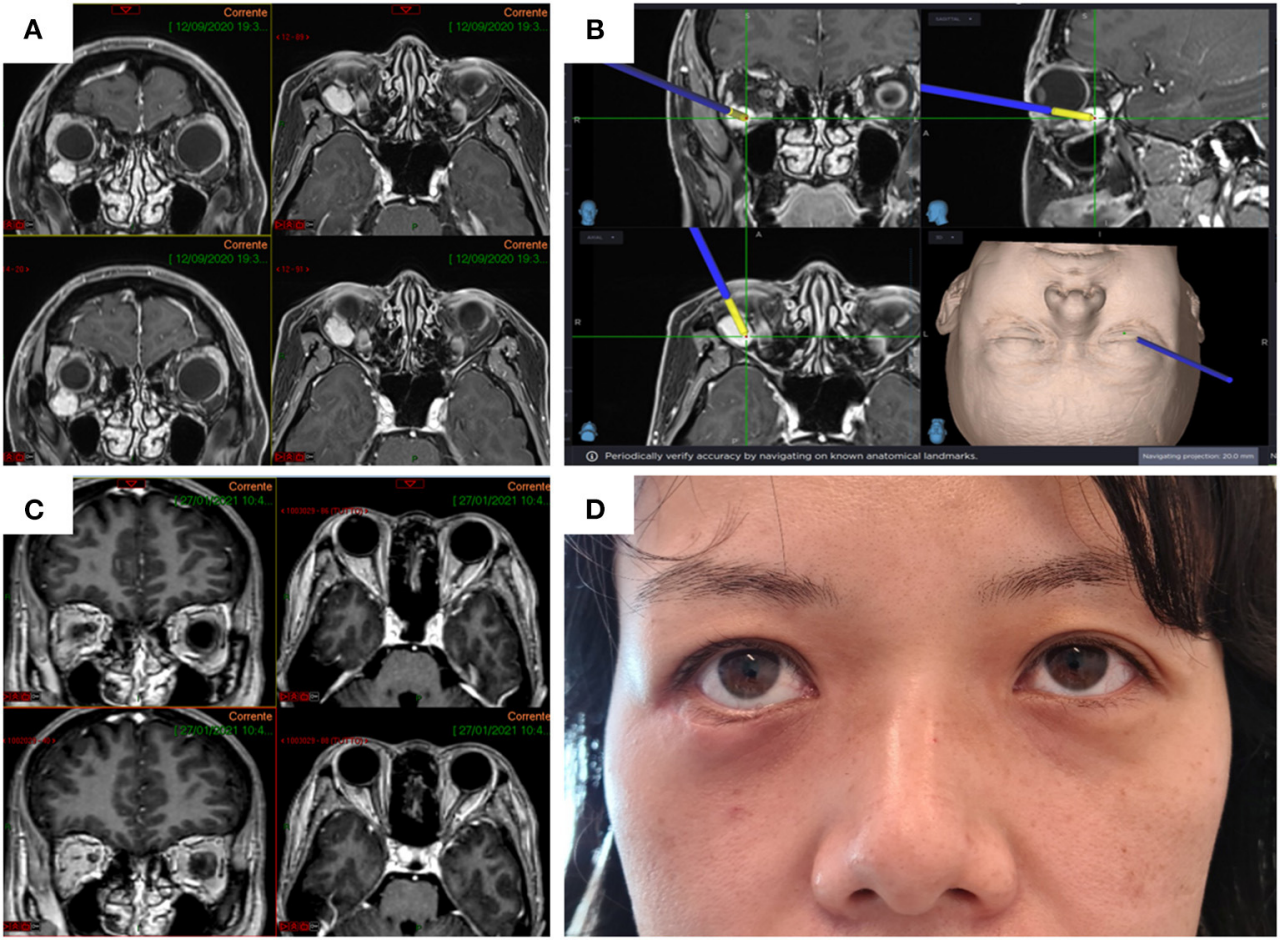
Preoperative enhanced MRI of the orbits (left, axial; right, coronal) showing the cavernous hemangioma located in the inferior lateral part of the right orbital intraconic space (A) and detail of tumor localization using navigation (B). Postoperative MRI showing complete removal of the hemangioma (C). The transpalpebral approach allows a minimally invasive access to the retro-orbital space ensuring excellent esthetic and functional results (D).
